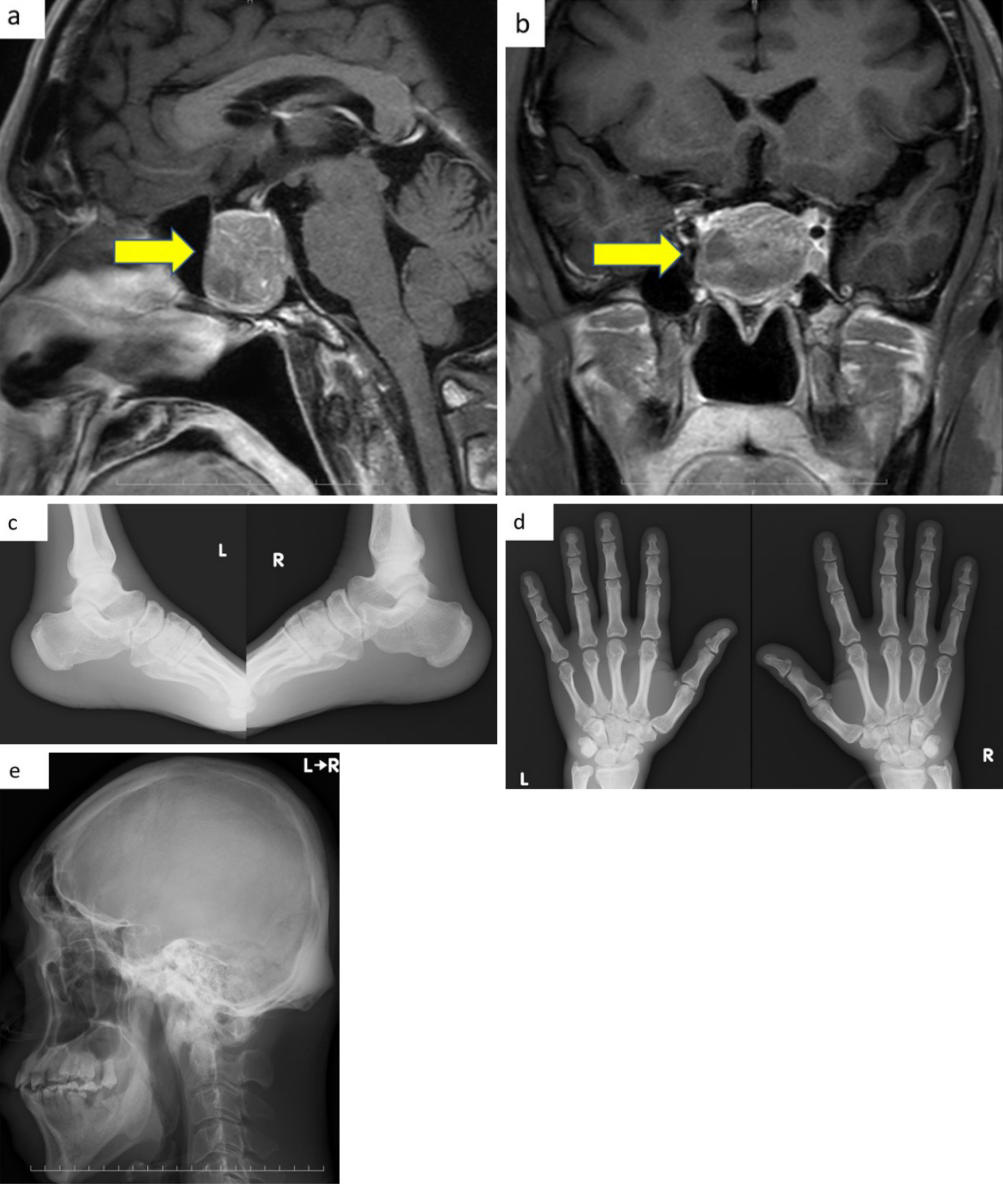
Contrast-enhanced head magnetic resonance image (T1-weighted image) shows a pituitary mass (arrow). (a) Sagittal section. (b) Coronal section Plain radiograph showing (c) heel pads (R 23 mm, L 25 mm); (d) cauliflower deformity; and (e) frontal sinus enlargement, sella turcica enlargement, mandibular protrusion, and malocclusion.
